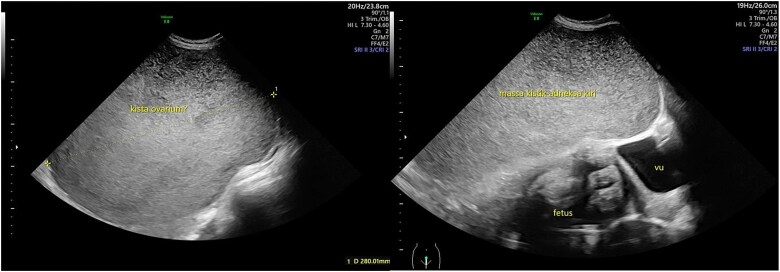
Ultrasound view of the cystic mass. The mass was unilocular without a solid part with a diameter of 28 cm.
